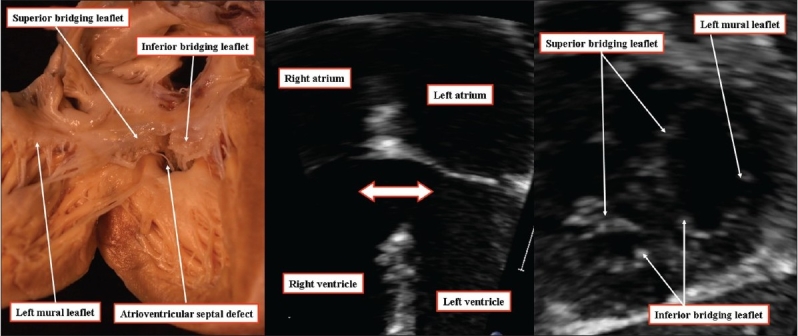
The anatomic image (left hand panel) and the echocardiograms (middle and right hand panels) are from different patients. They show how an atrioventricular septal defect with common atrioventricular junction can be arranged to permit only ventricular shunting when the bridging leaflets of the common atrioventricular valve are fused to the leading edge of the atrial septum. The right hand panel, in the short axis, confirms the trifoliate arrangement of the left atrioventricular valve
